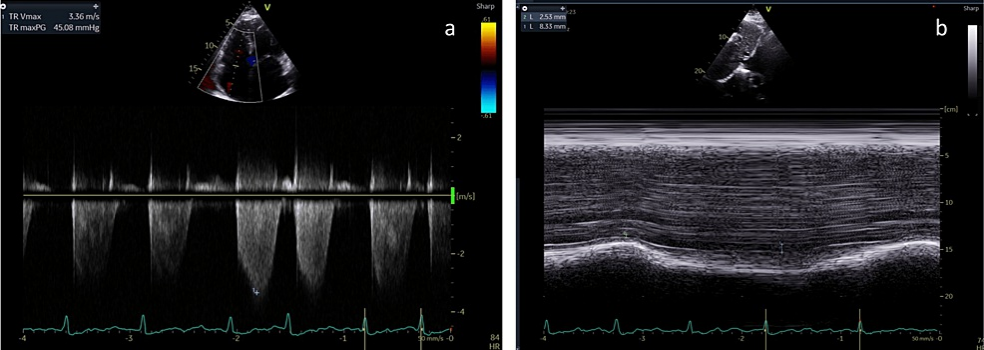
Estimated pulmonary arterial systolic after Graves' treatment Echocardiographic parameters, evaluated after Graves’ treatment and TRAbs levels decrease, showing an estimated pulmonary arterial systolic of 55 mmHg. Systolic artery pressure was estimated from a) tricuspid regurgitant jet velocity of 3.36 m/s and calculated by Bernoulli equation maximum gradient of 45 mmHg plus b) estimated right atrial pressure of 10 mmHg (slightly dilated inferior vena cava with respiratory variation > 50%).
